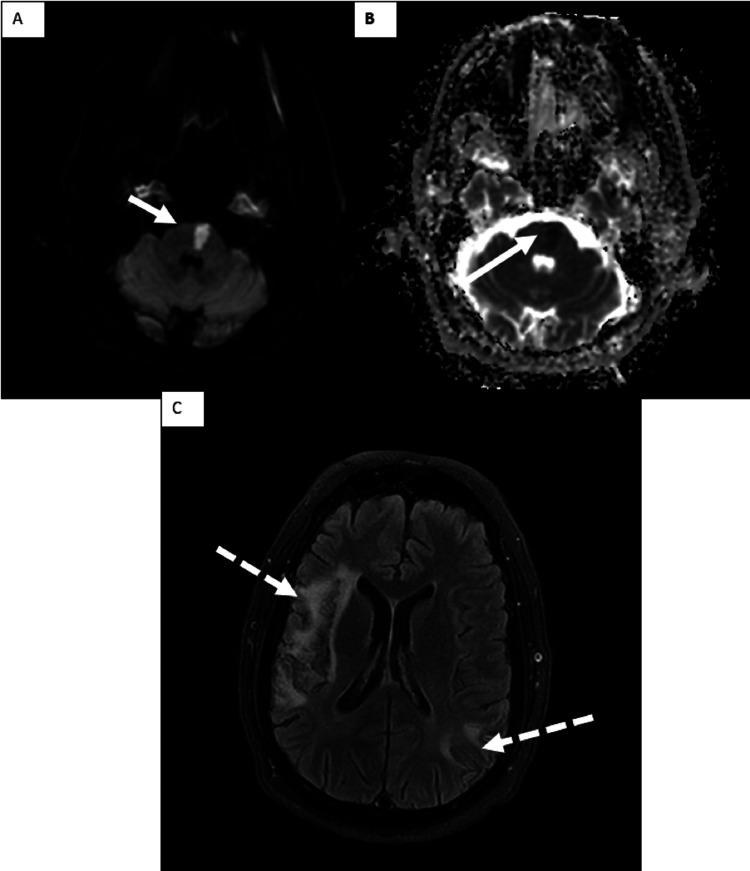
Axial MRI images (A,B) DWI and ADC showing an acute left pontine infarct (white arrow) (C) FLAIR sequence showing bilateral chronic infarcts in the right middle cerebral artery territory (right frontal operculum and right temporal lobe with a peripheral watershed infarct in the left parietal-occipital junction) (two dashed arrows) DWI, diffusion-weighted image; ADC, apparent diffusion coefficient
